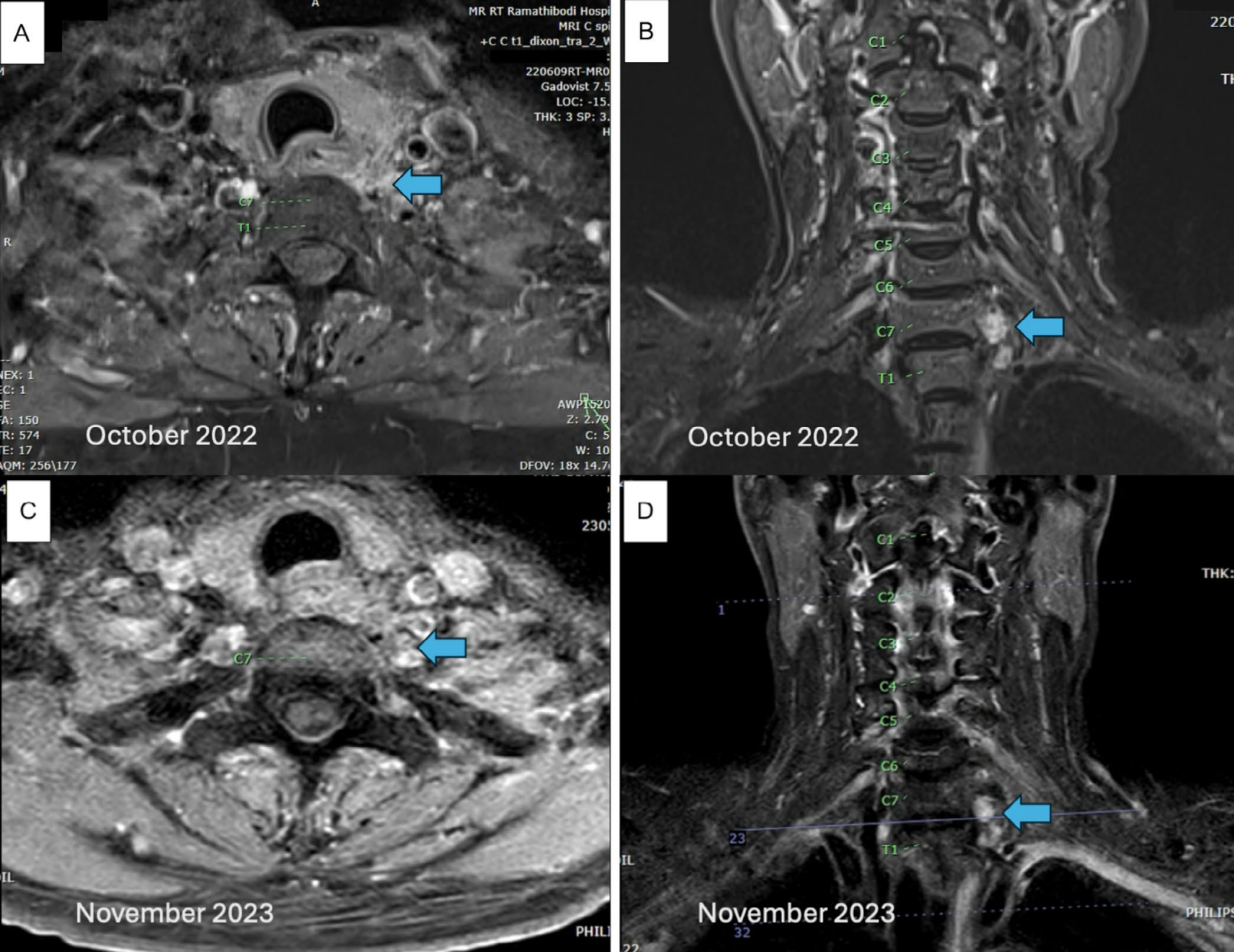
Six‐month follow‐up MRI showing a T1‐isointense, T2‐hyperintense lesion along the inferior border of the left C7 vertebral body, suggestive of residual tumor. (A) Axial T1‐weighted and (B) Coronal T2‐weighted images from October 2022. Follow‐up MRI from November 2023 shows no significant change in the 0.9 cm lesion, suggesting either residual tumor or post‐treatment changes: (C) Axial T1‐weighted and (D) Coronal T2‐weighted images.
